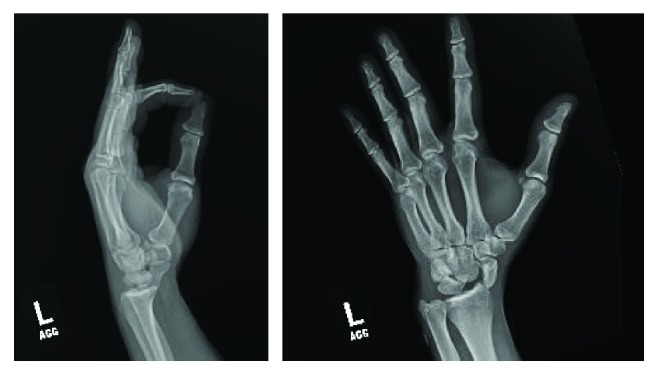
Presenting plain films demonstrating volar scaphoid dislocation.
